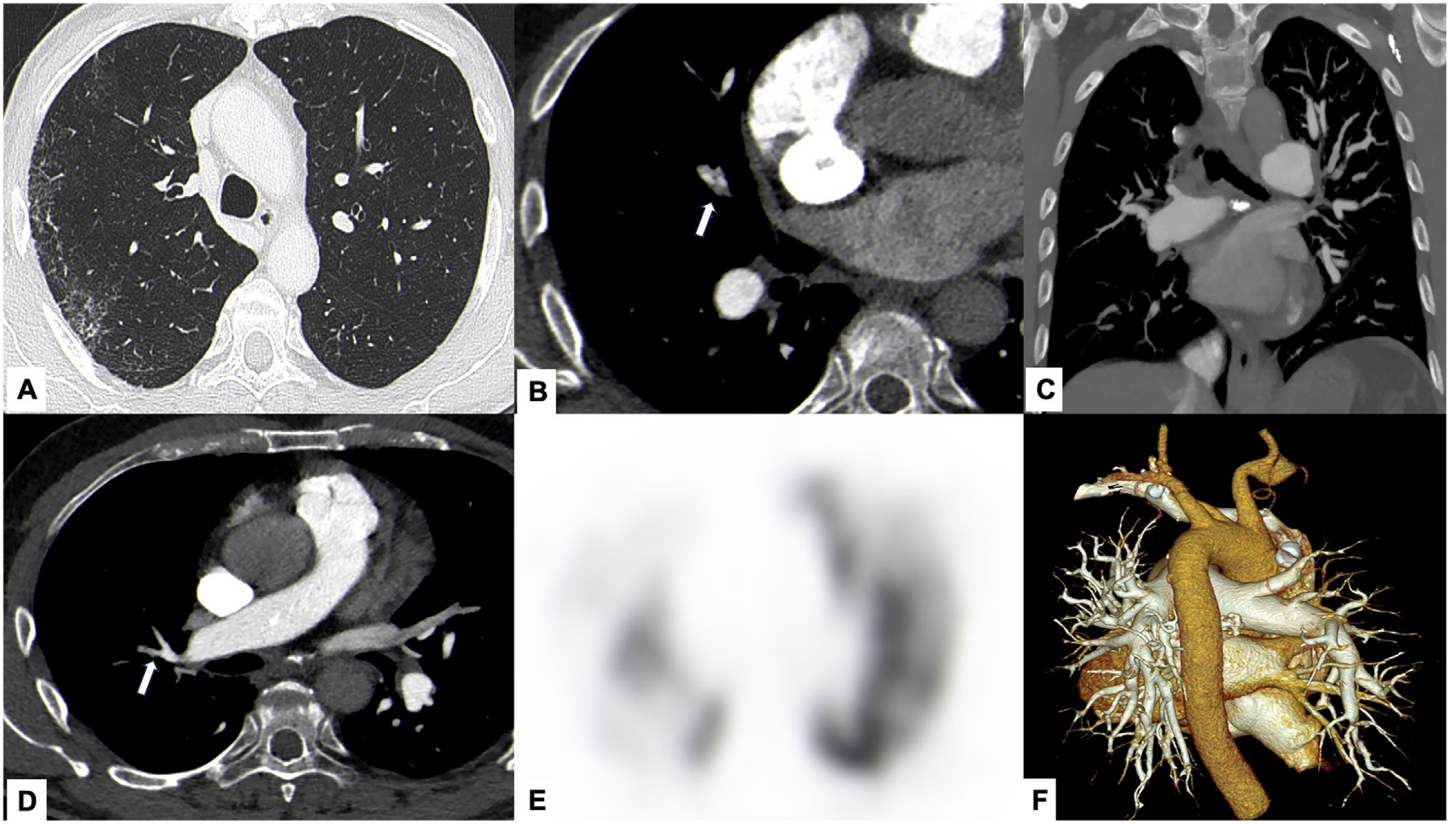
Case 2 – Chronic pulmonary thromboembolism (CPTE) with secondary unilateral pulmonary fibrosis.* CT pulmonary angiography demonstrates narrowing of right upper lobe pulmonary arteries with eccentric thrombotic material and endoluminal webs (arrows in A, B), consistent with CPTE. Mild unilateral interstitial thickening is visible in the dorsal portion of the right upper lobe (arrows in C). MIP reformatting (D) and VRT 3D reconstruction (E) highlight decreased vascular branching in the affected region. Pulmonary scintigraphy (F) confirms reduced perfusion of the right lung, supporting the diagnosis of chronic right CPTE.
